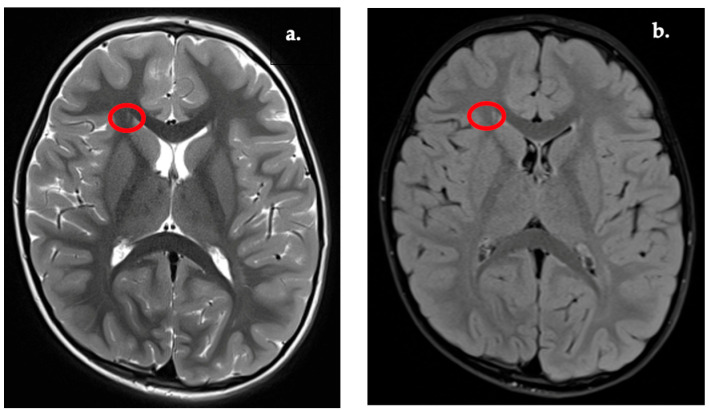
WMSA of the lateral ventricles (frontal horns). (a) T2w image. (b) FLAIR image.
